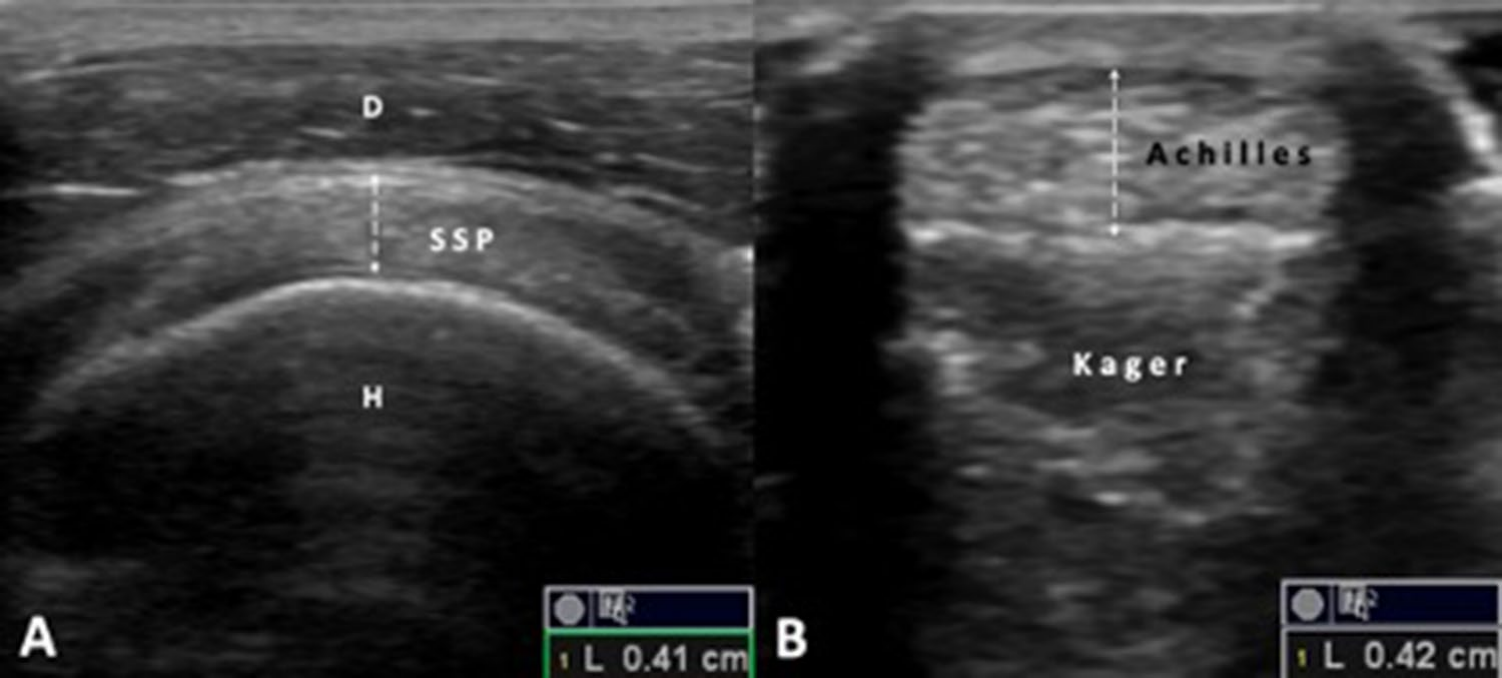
Ultrasonographic measurements of the supraspinatus (SSP). A and Achilles B tendons during axial imaging. *H* humerus, *D* deltoid muscle, Kager: fat pad
